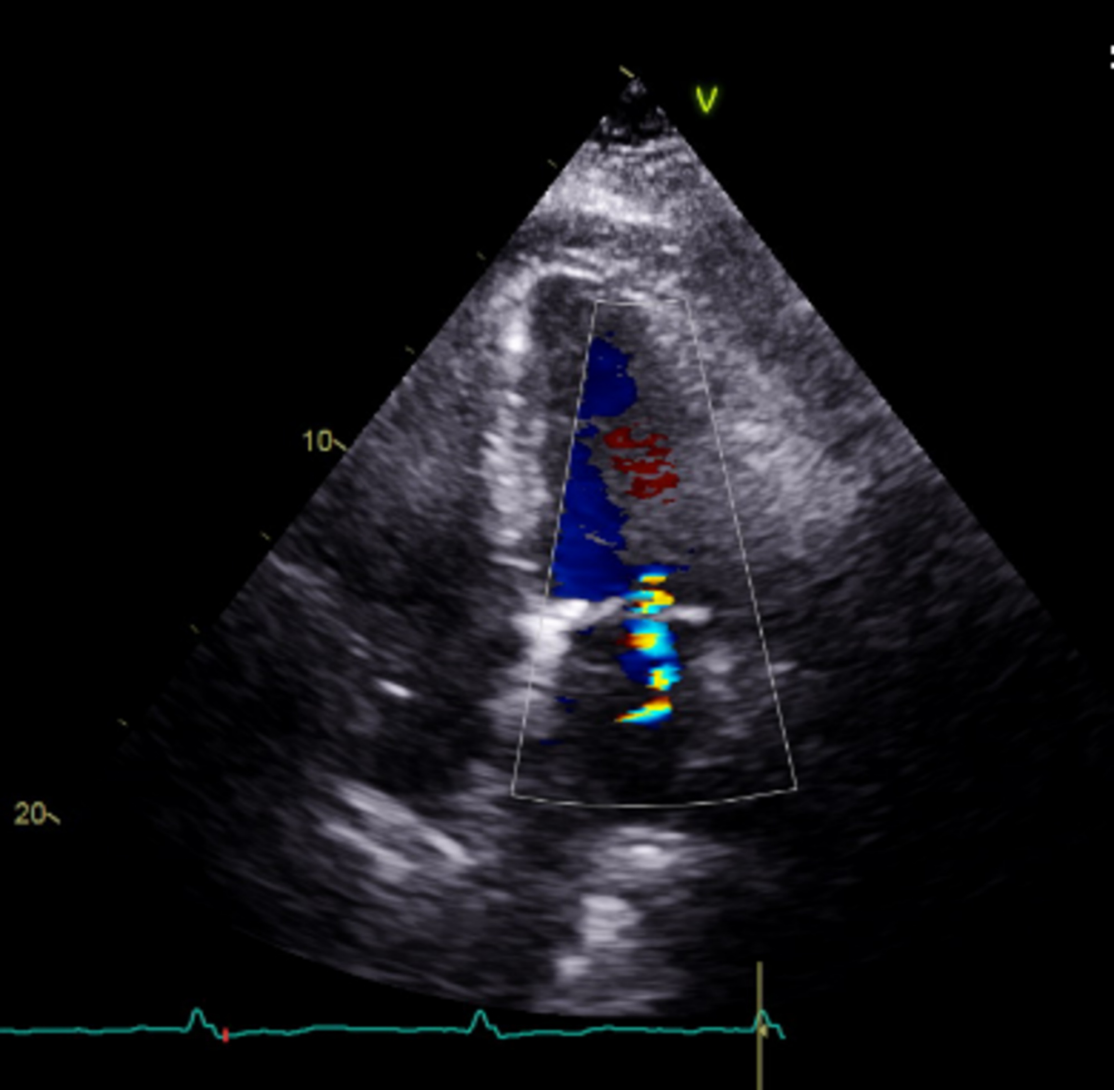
An echocardiogram showing an apical four chamber view There is normal appearance of the myocrdium, no evidence of infiltrative diseases, ischemic changes or scars. There is only mild mitral regurgitstion shown in the image.
